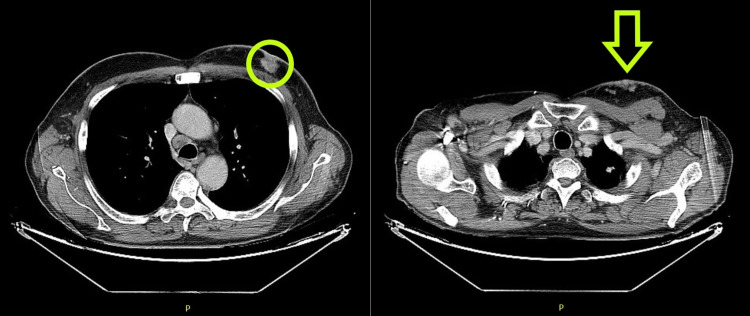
Chest CT on the left showing primitive breast cancer (green circle) and on the right demonstrating skin metastasis (green arrow) CT: computed tomography
